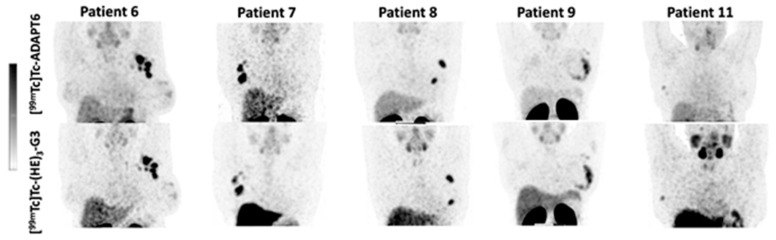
Comparison of SPECT images (maximum intensity projections) obtained using [^99m^Tc]Tc-ADAPT6 (2 h after injection) and [^99m^Tc]Tc-(HE)_3_-G3 (4 h after injection) in patients with HER2-positive breast cancer. The upper setting of a linear intensity scale is adjusted to SUV 6.8 in all images.
